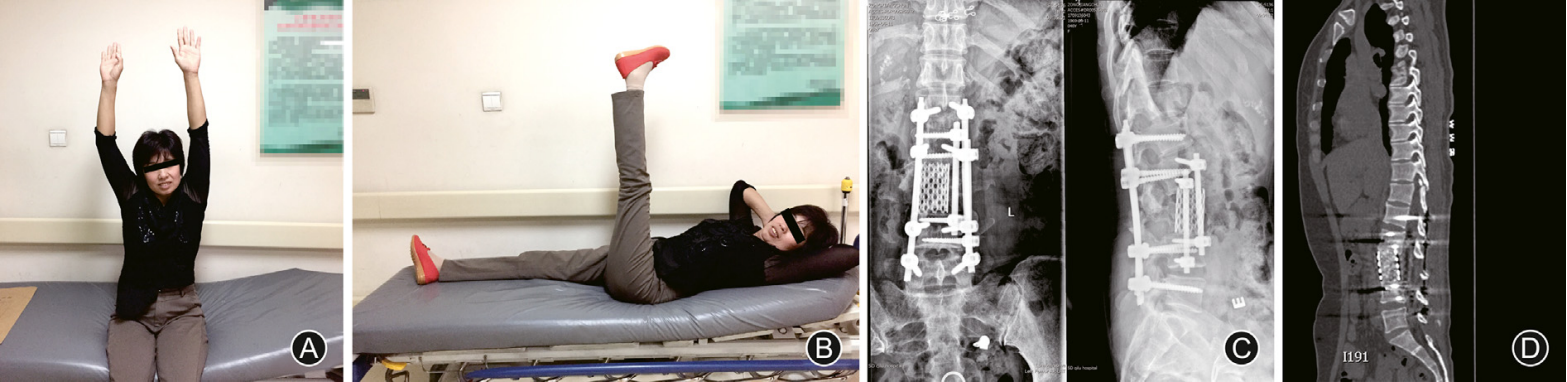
Rehabilitation functions at postoperative 6-month follow-up. At postoperative 6-month, this patient was identified to have incomplete neurological improvement (A and B). Postoperative radiography plain (C) and CT scan (D) demonstrated a stable spinal alignment.
